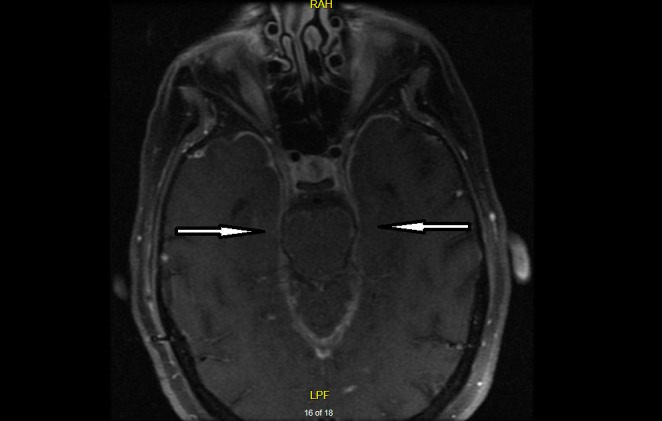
MRI of the brain with contrast showing widespread leptomeningeal enhancement (arrows) raising suspicion for leptomeningeal carcinomatosis.
